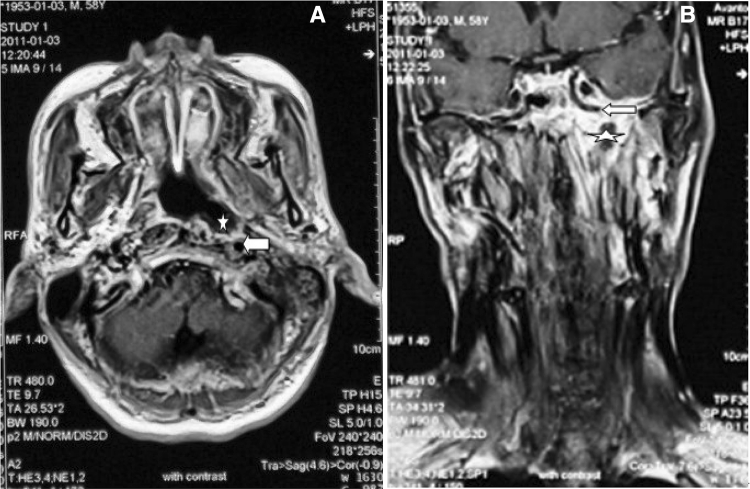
Images of a 30-year-old man (number 7 in the table) who received 132 Gy to treat a primary tumor; intractable epistaxis occured 6 months after radiotherapy (RT). a, b Horizontal and coronal position of MRI shows the pharyngeal recess (*pentagon*) and petrous portion of the internal carotid artery (ICA) (*arrowhead*)
